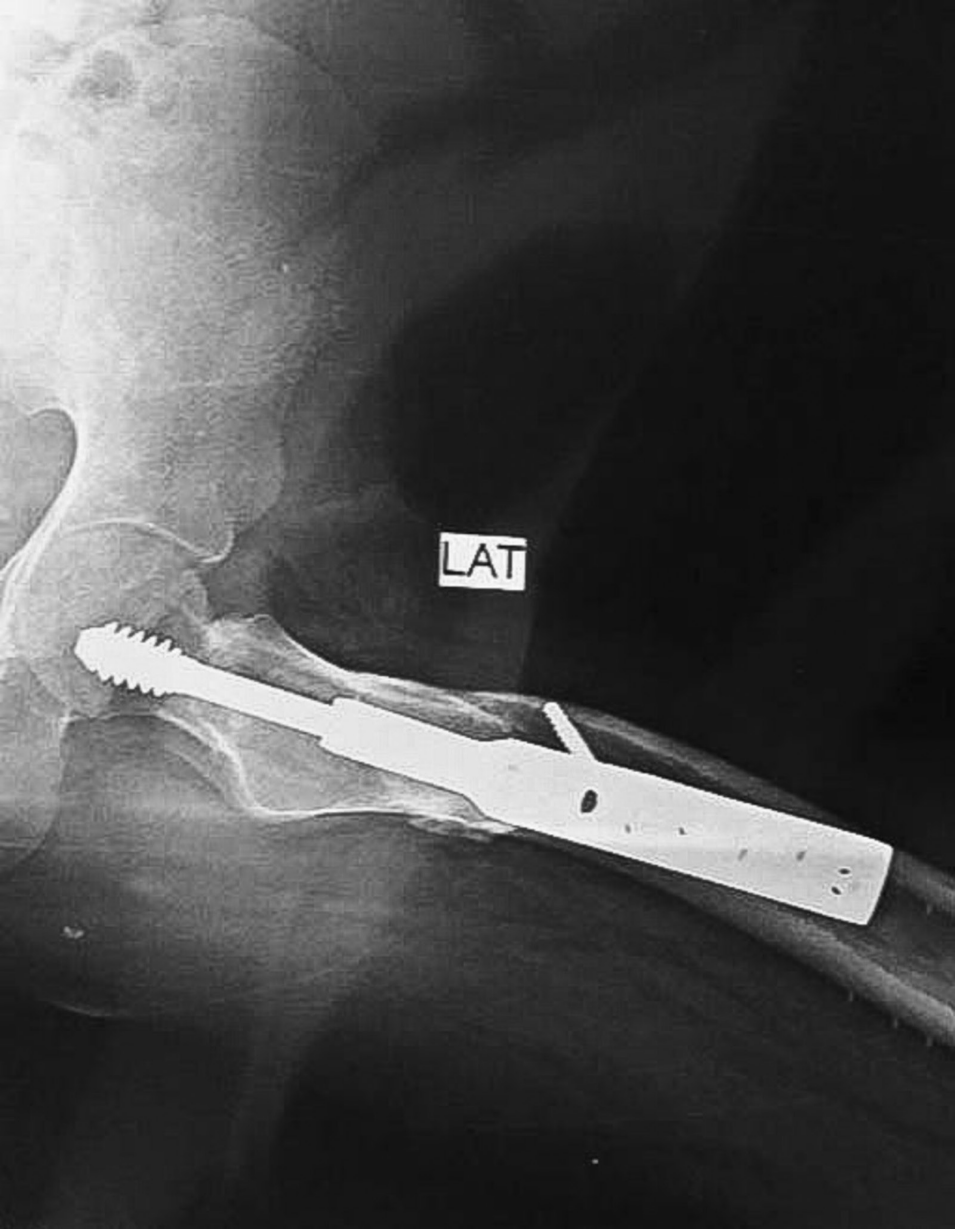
Lateral radiograph of the patient after 2 years, postoperativerly, showing a well healed fracture and osteotomy sites.
